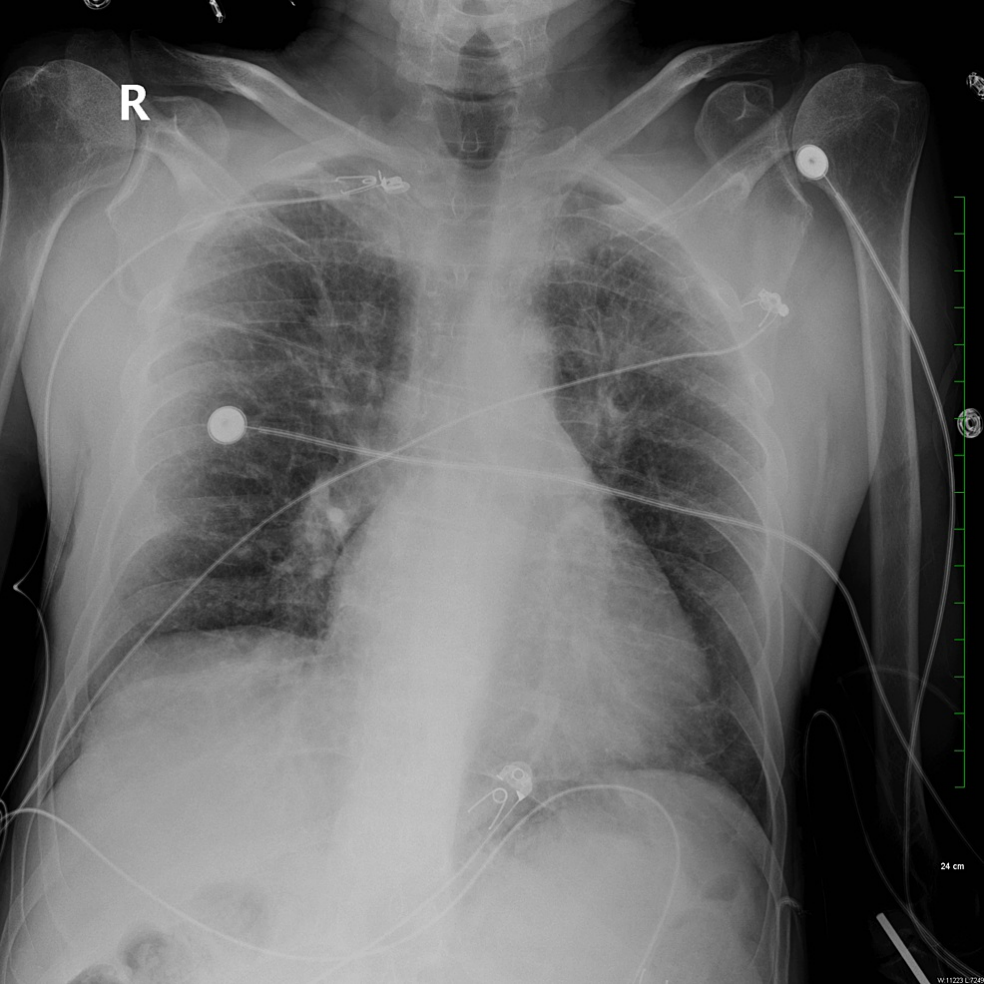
Chest X-ray revealing interstitial coarsening in the upper lung fields, accompanied by mild hyperexpansion upon hospital admission.
